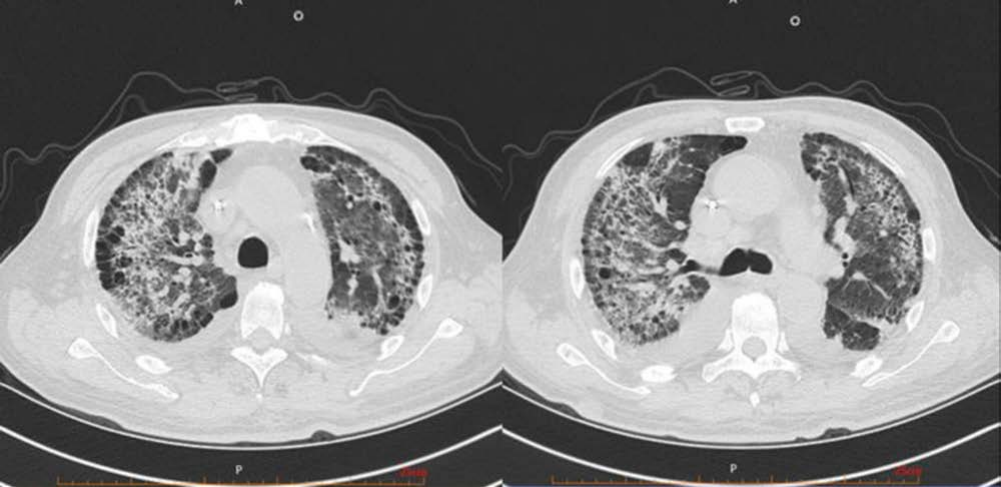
HRCT of the lung on April 11th: compared with April 2nd, multiple exudative lesions in both lungs are similar to those observed previously. HRCT = high-resolution computed tomography.
